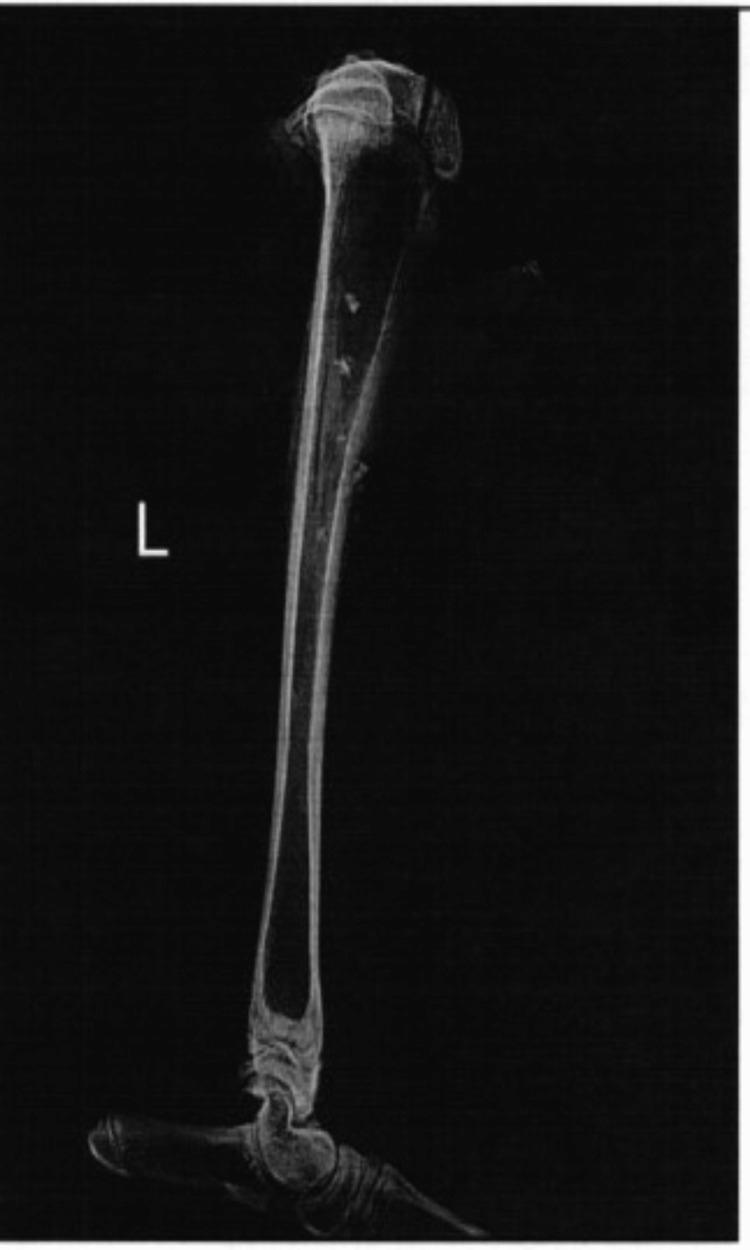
Mediolateral radiographic view 120 days after implantation. The bone defect was filled and new bone cells were organized.
